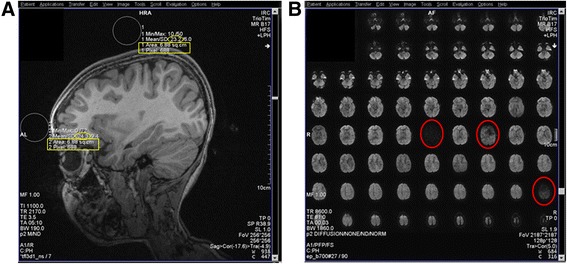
Objective motion quality assurance protocol. a Depicts ROI placement for T1-weighted assessment. The size of the ROI was between 650 and 850 pixels or 6.50 and 8.50 cm^2^ (*yellow rectangle*). The ratio of the mean signal intensity (*yellow ovals*) anterior/superior ROIs was required to be ≤2.0. b Depicts an example of slice dropout (*red circles*) in one volume of a diffusion-weighted scan. Volumes were excluded if any slice dropout was present, and the number of excluded volumes was required to be ≤5 out of a total of 30 volumes
